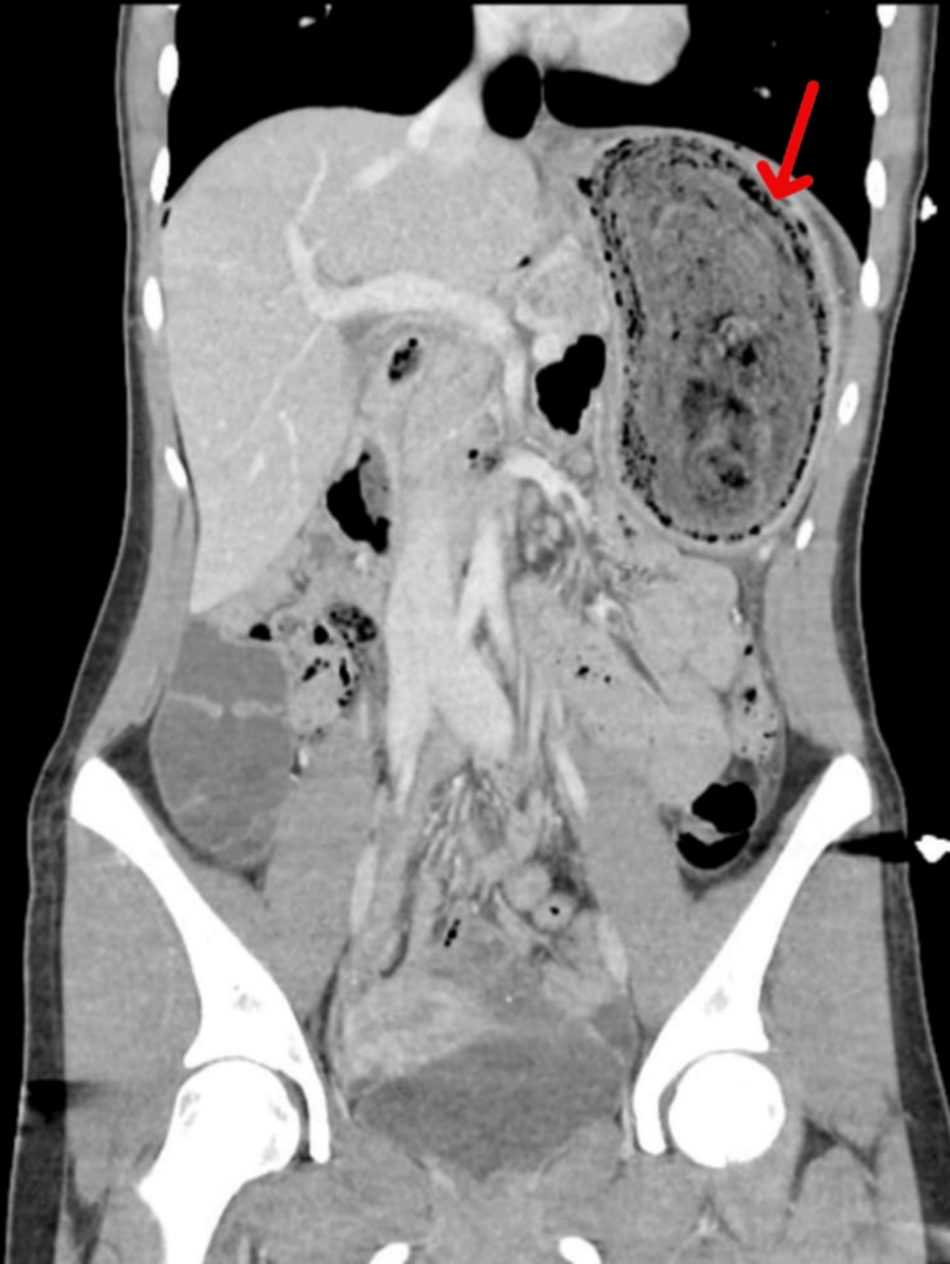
CECT whole abdomen (coronal section) showing heterogeneous intraluminal mass in the stomach extending to the proximal jejunum, with a mottled gas pattern (Red arrow). CECT: Contrast-enhanced computed tomography
